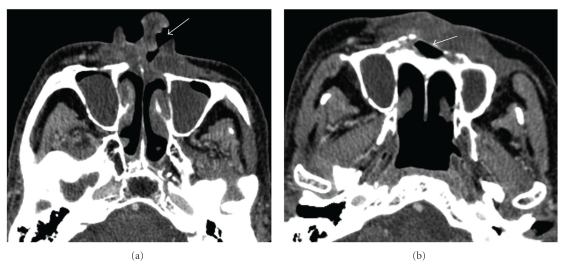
Orocutaneous fistula: (a, b) Axial contrast-enhanced CT of the oral cavity (soft tissue window) shows an orocutaneous fistula involving the left nasal ala (arrow) and extending through the maxilla (arrow) towards the oral cavity.
